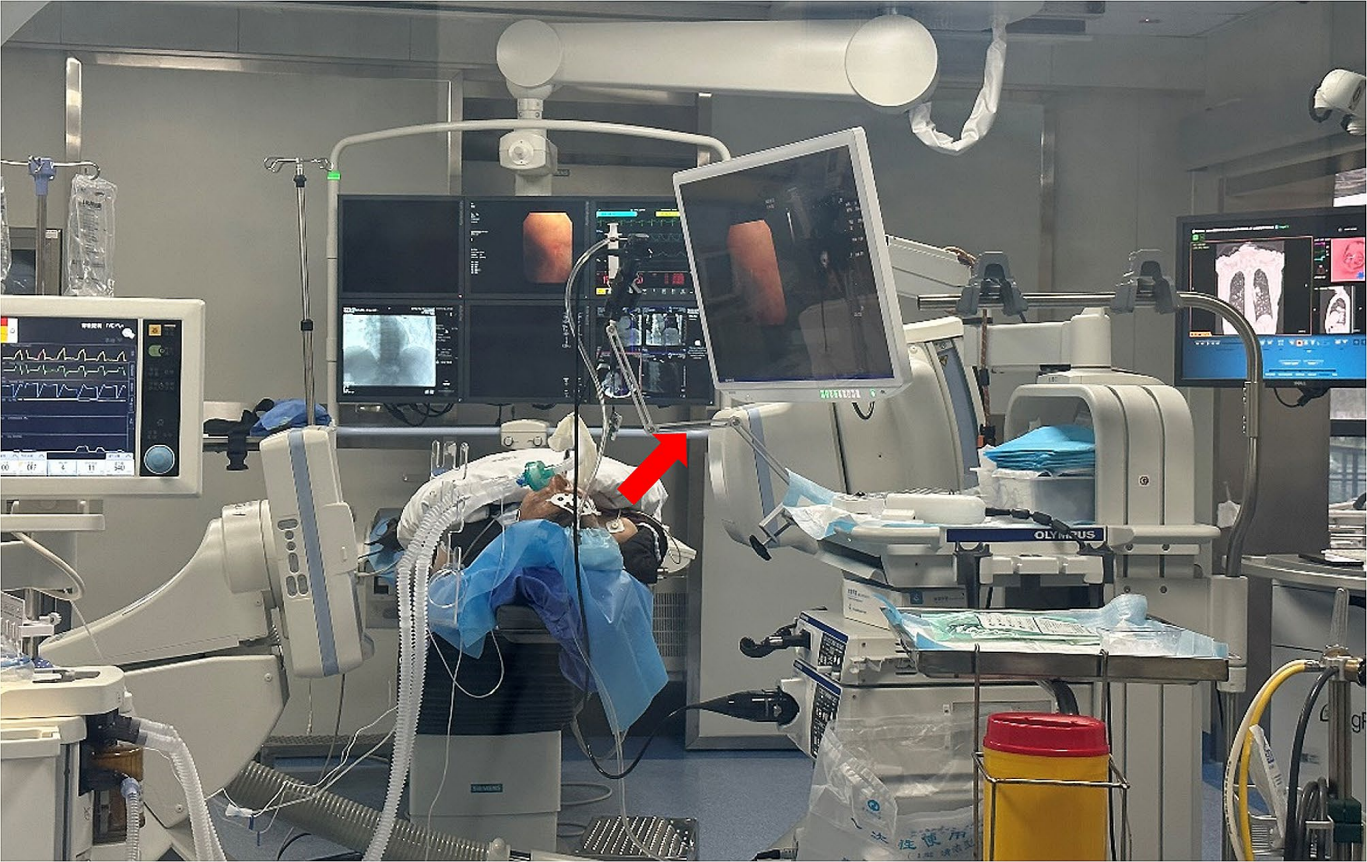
Room set up with fixed c-arm. The red arrow corresponds to the arm that holds the bronchoscopy when performing the CBCT scan
